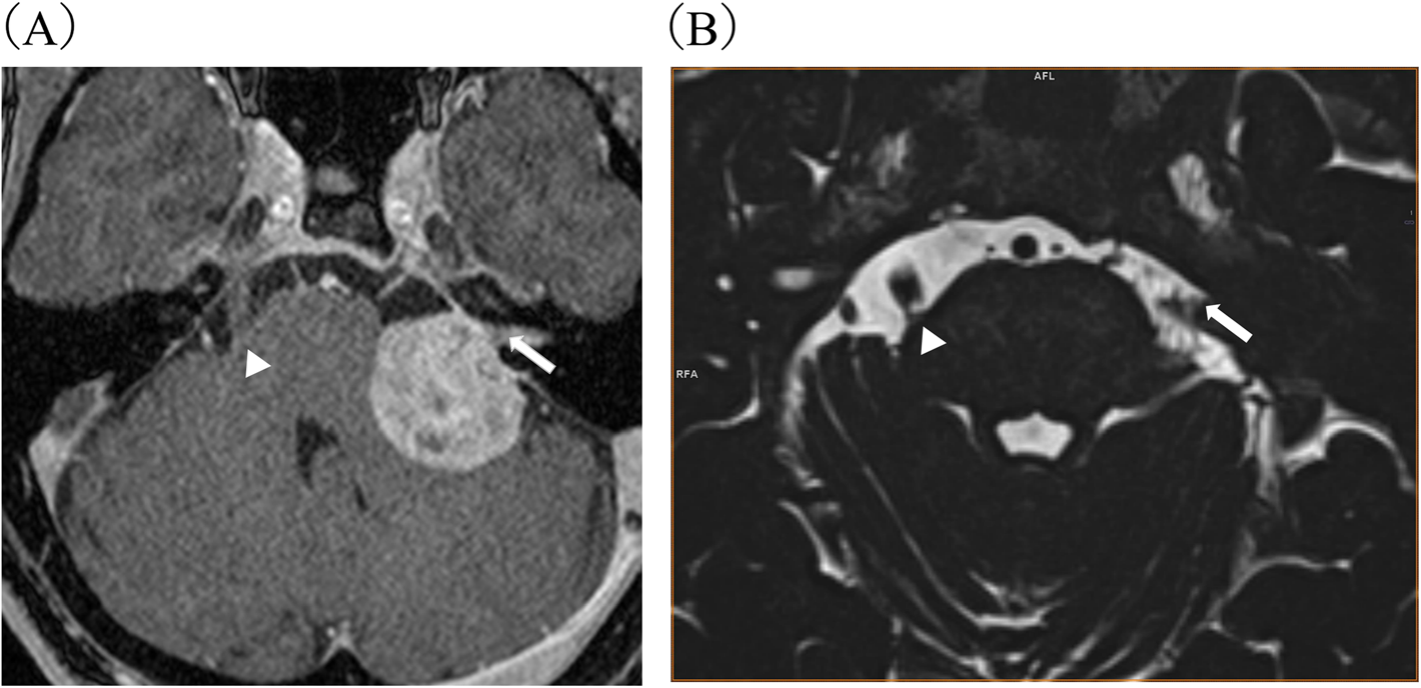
(A) Magnetic resonance imaging scans of VS (4 years before the patient’s first visit to our clinic) (well-defined mass involving the left cerebellopontine angle) (white arrow: vestibular schwannoma affecting the left trigeminal nerve, arrow head: right trigeminal nerve) (B) Magnetic resonance imaging scans from the postoperative period (white arrow: left trigeminal nerve, arrow head: right trigeminal nerve)
